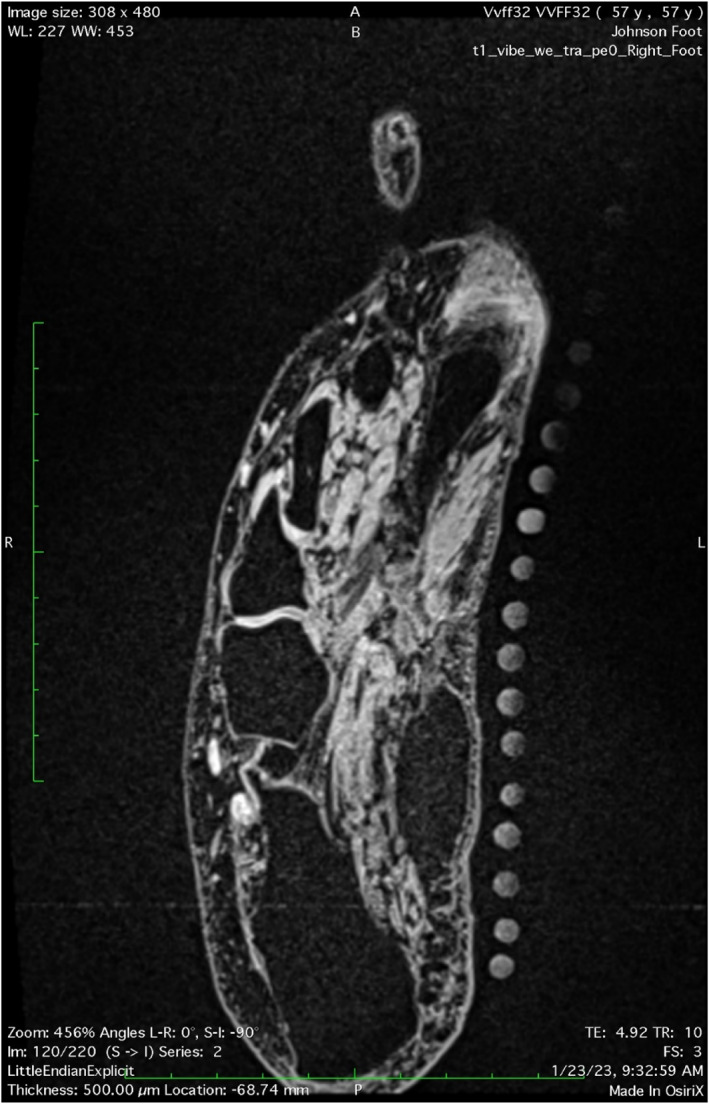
Fitted gel capsule strip on MRI.
